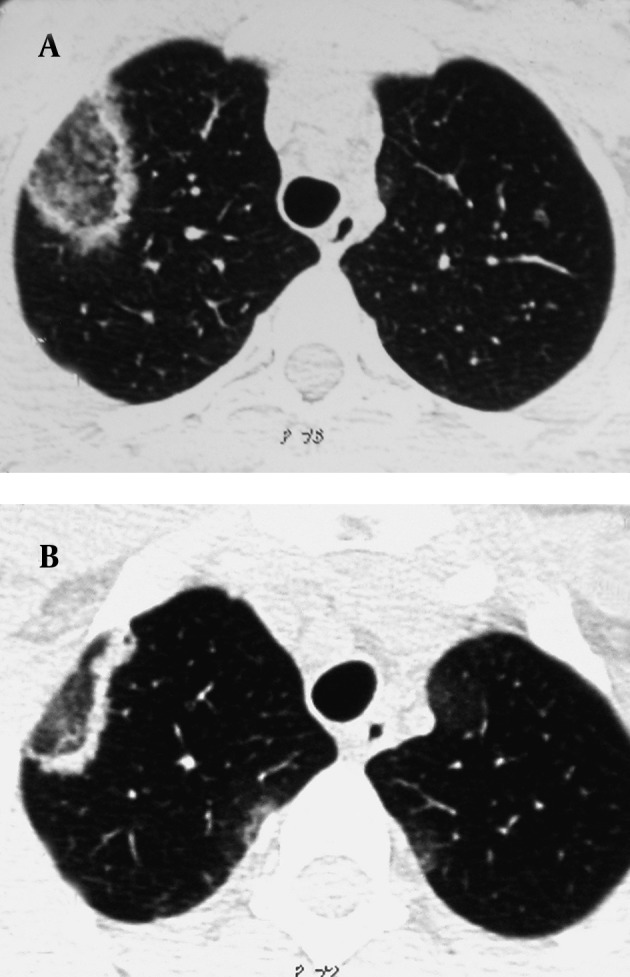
A, B) Axial CT images at the level of great vessels show opacity with RHS appearance
